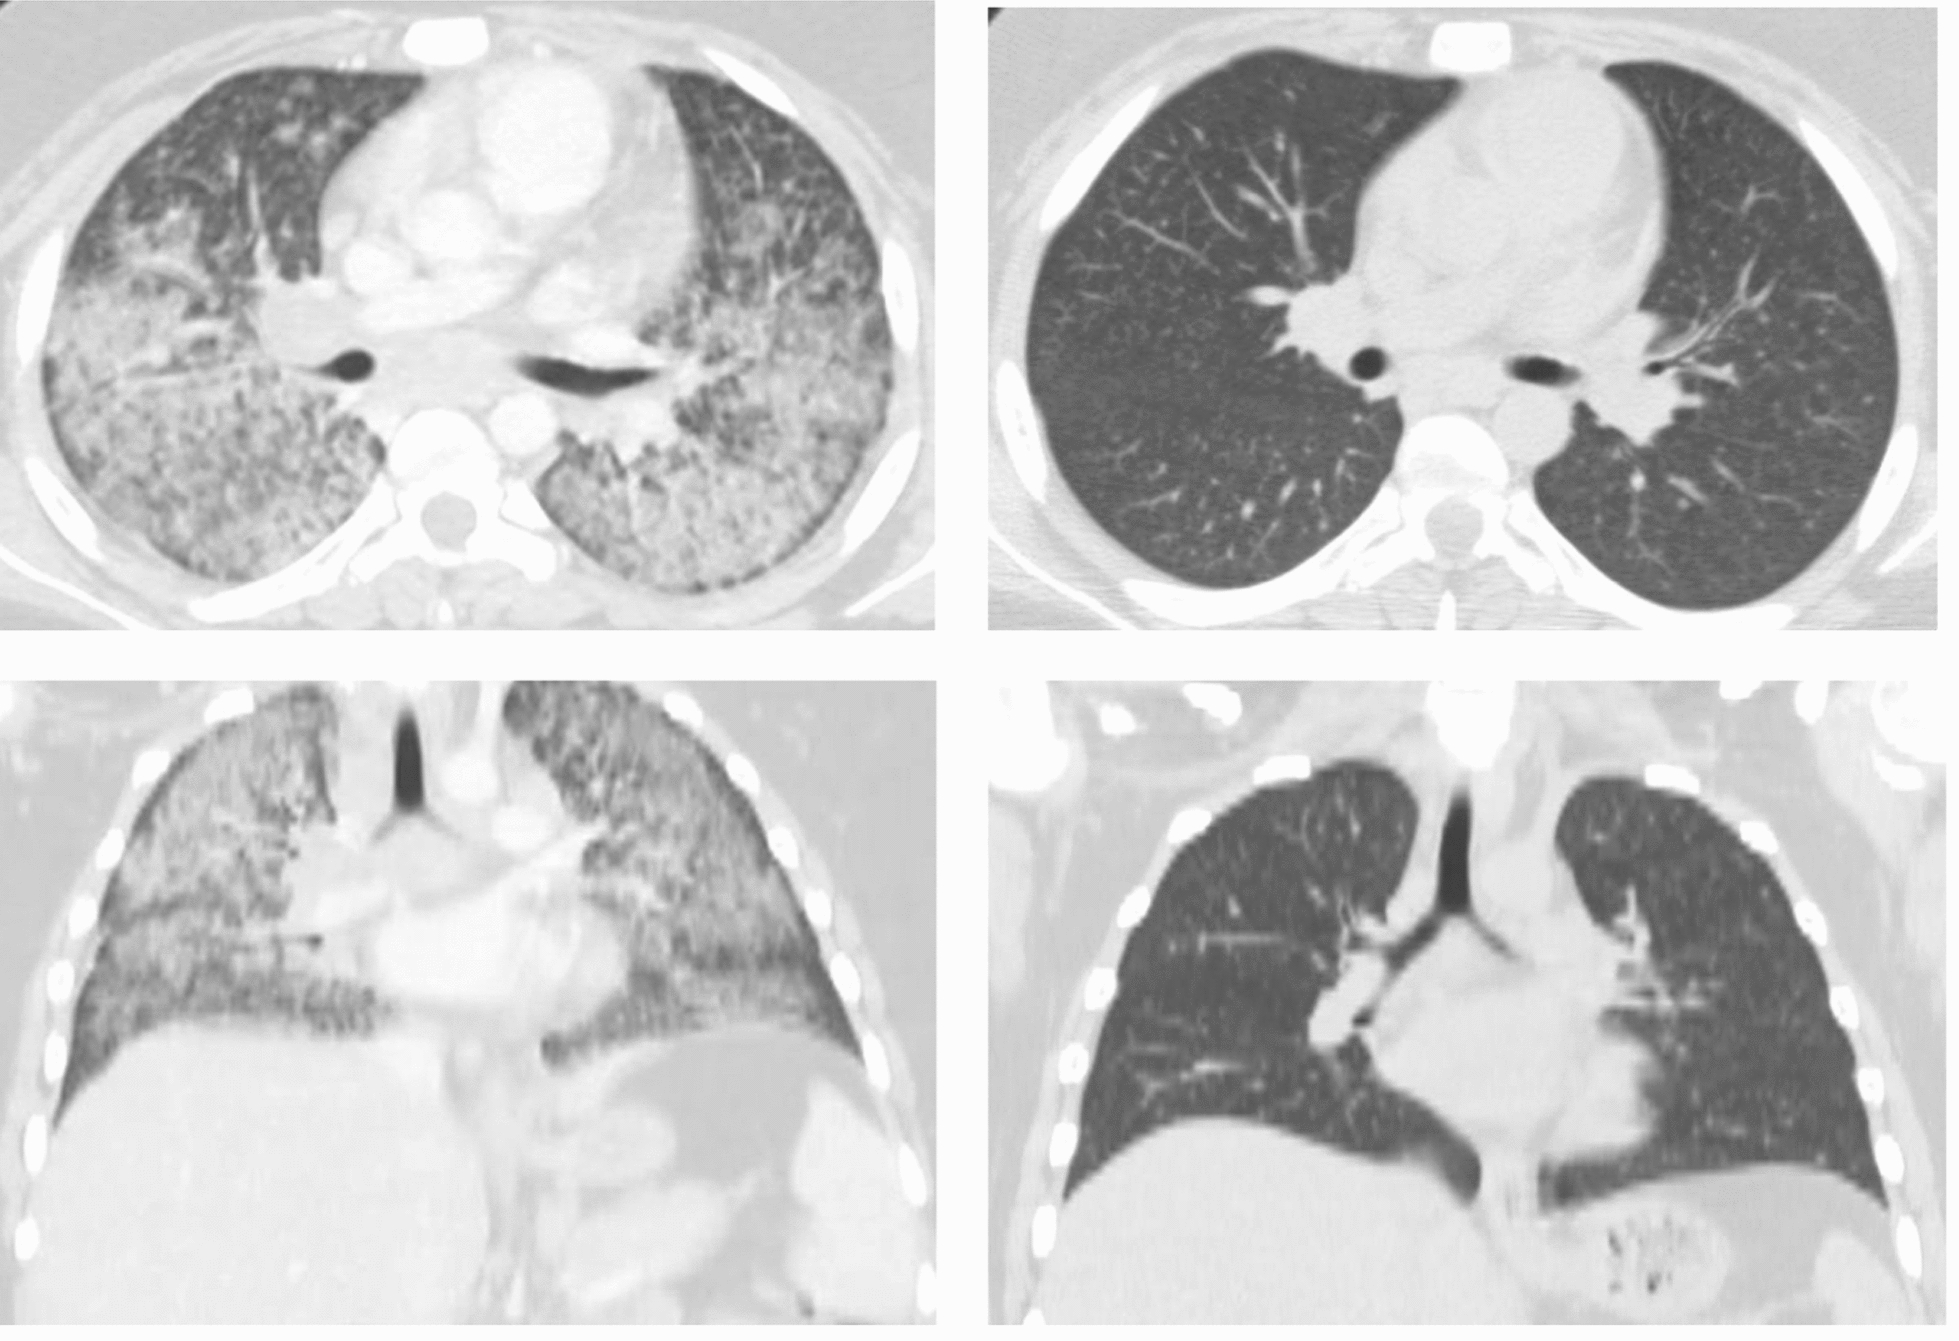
Computed tomography (CT) of the chest in axial (top) and coronal (bottom) planes. On admission, initial CT (left) showing extensive pulmonary disease. Repeat CT (right), obtained after inpatient liposomal amphotericin B and outpatient posaconazole therapy were completed, showing resolution of the infiltrative pulmonary disease
